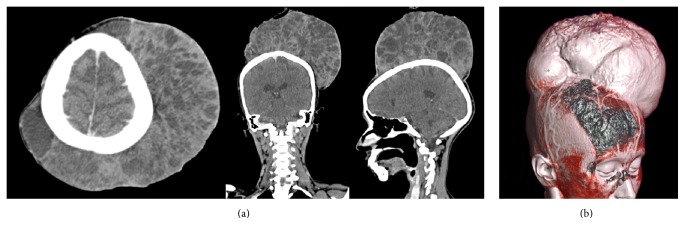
Computed tomography (CT) scan with 3D reconstruction showing highly vascular tumour with clean surgical plane with underlying calvarium.
